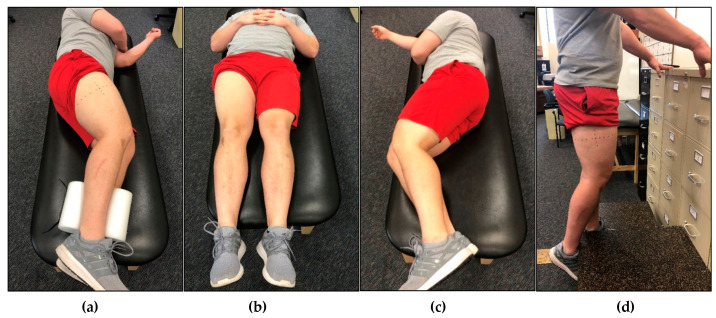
An example participant in different rest positions prior to ultrasound analysis of the vastus lateralis (VL) muscle. (a) Participant laying in the non-dominant lateral recumbent (NDLR) position with the dominant limb exposed. This was the position that all ultrasound images were captured in (except for standing), however, the rest position utilized beforehand differed. The participant utilized this rest position for immediately post (IP) analysis and rest for 15 min in the NDLR position. (b) Participant laying in the supine (SUP) position. After 15 min in this position, the participant was instructed to flip over to the NDLR position, and ultrasound images were captured immediately following. (c) Participant laying in the dominant lateral recumbent (DLR) position with the dominant leg compressed. After 15 min in this position, the participant was instructed to flip over to the NDLR position, and ultrasound images were captured immediately following. (d) Participant in the standing (ST) position. The ultrasound images were captured while the participant remained standing. Participants were instructed to bear weight on the non-dominant leg while resting the dominant leg against a platform to allow for a bend in the knee. Participants were instructed to stand for 15 min in between each position.
